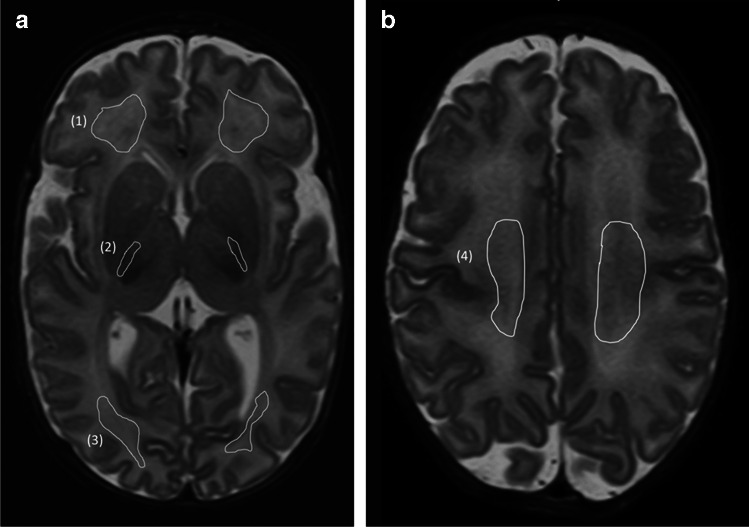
Representative axial synthetic reconstructed T2 short tau inversion recovery magnetic resonance images at the level of the basal ganglia (a) and the centrum semi-ovalae (b) demonstrate manually delineated regions of interest (ROIs) in a girl scanned at a postmenstrual age of 40 weeks. There is abnormally prolonged T1 (1582 ms) and T2 (141 ms) relaxation times in the posterior limb of the internal capsule above the defined cutoff thresholds, indicating impaired development. This girl exhibited impaired development at 2 years, despite conventional MRI showing no abnormalities. *1* frontal white matter; *2* posterior limb of the internal capsule; *3* parietal white matter; *4* central white matter of the centrum semiovale
